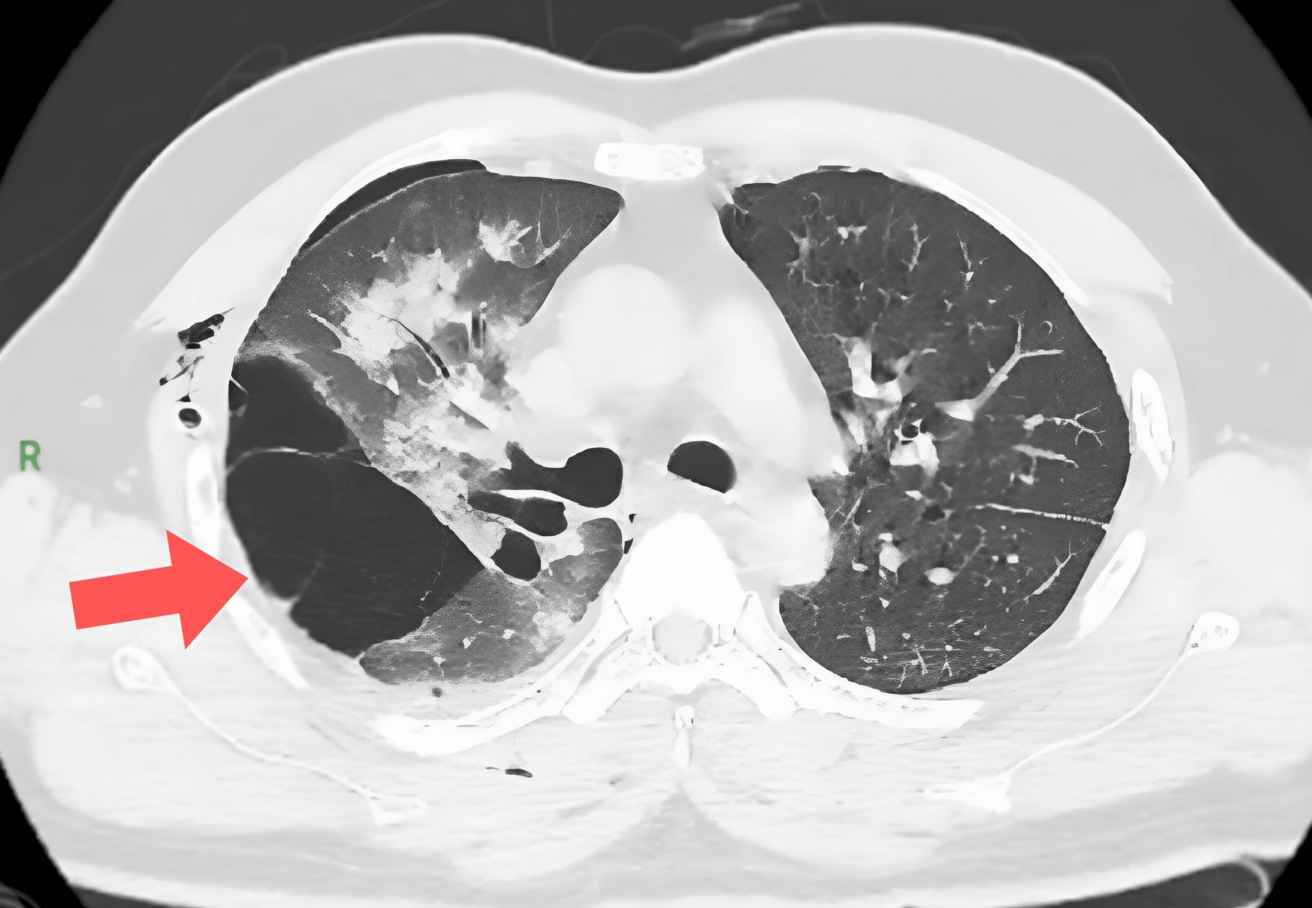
High-resolution computed tomography (HRCT) thorax showing lung cysts in a patient with Birt-Hogg-Dubé (BHD) syndrome (arrow indicating a large pulmonary cyst in the lung parenchyma).
